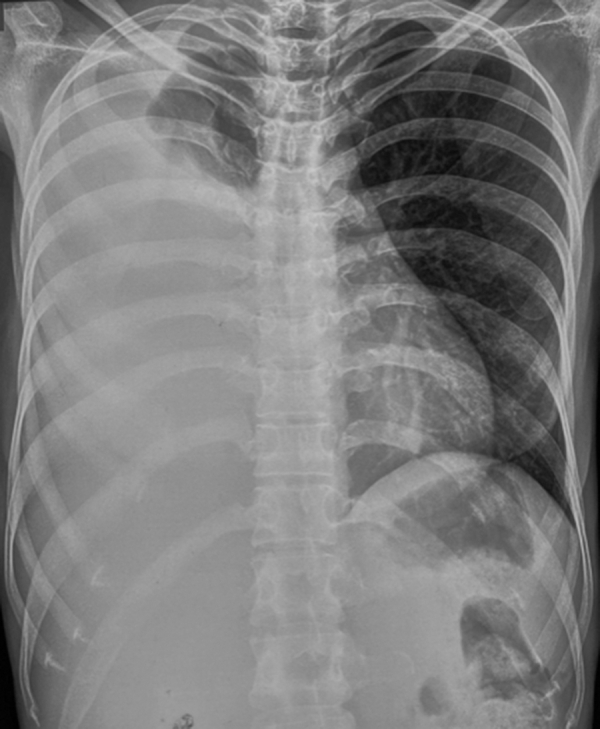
Chest radiograph posteroanterior view shows an opaque right hemithorax with a contralateral shift of the mediastinum.
